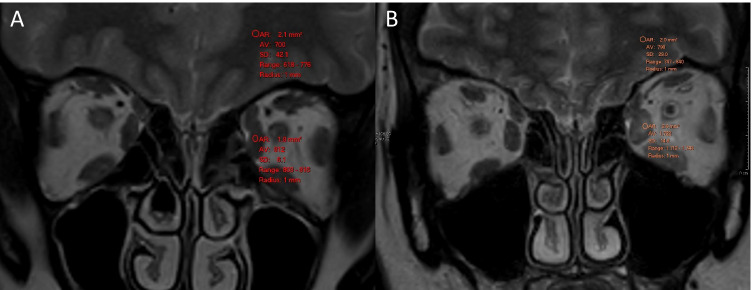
Signal Intensity ratio. Normal coronal T2-weighted MRI (A) showing the signal intensity ratio of the extraocular muscle and brain white mater. Coronal T2-weighted MRI (B) in a patient with active TED involving the left inferior and medial rectus muscles showing the signal intensity ratio between the inflamed left medial rectus and brain white mater
